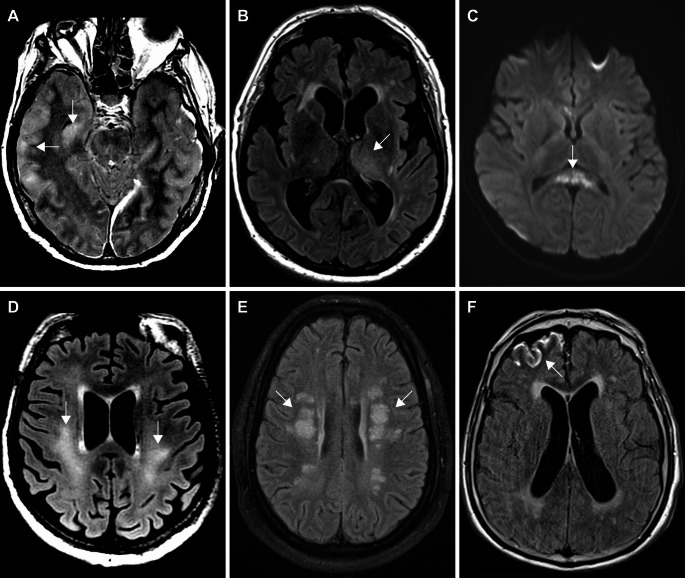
Non-stroke patterns: Axial FLAIR (a, b, d, e), post-contrast axial FLAIR (f), axial diffusion-weighted (c) MR images. A: cortical lesions involving the right hippocampus and right lateral temporal cortex lesions (arrowheads; pattern A1); b: left thalamic lesion (arrowhead; pattern A2); c: Cytotoxic lesion of the corpus callosum (CLOCC) (arrowhead; pattern B1), showing restricted diffusion; d: extensive, ill-defined, and confluent supratentorial white matter lesions (arrowheads; pattern B2); e: circumscribed and multifocal white matter lesions (arrowheads; pattern B3); f: right frontal leptomeningeal enhancement (arrowhead; pattern C)
